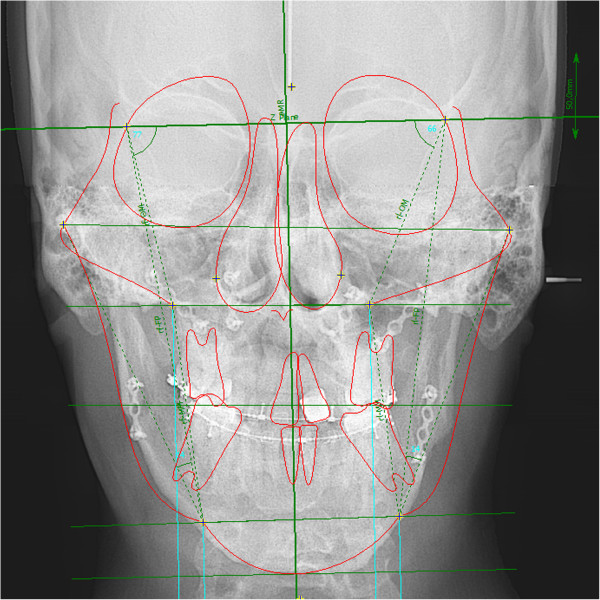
Postoperative frontal cephalometric x-ray shows that the facial symmetry has improved significantly.
